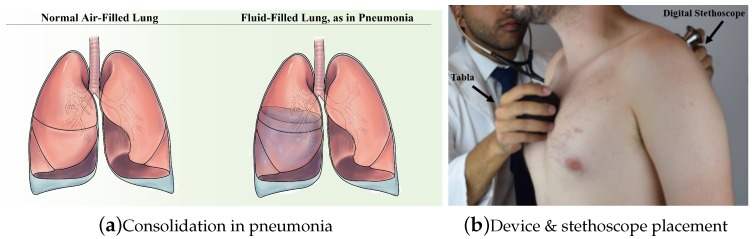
(a) a lung with consolidation characteristic of pneumonia and a healthy lung. Pneumonia leads to the accumulation of protein-rich fluid (exudate) in the lungs. The presence of exudate can be detected using different techniques, e.g., chest X-rays and acoustic analysis; (b) the Tabla is placed on the sternum and the stethoscope is moved to different quadrants of the lungs to record audio data, which is transmitted via Bluetooth to a smartphone (not shown). Figure from [22].
